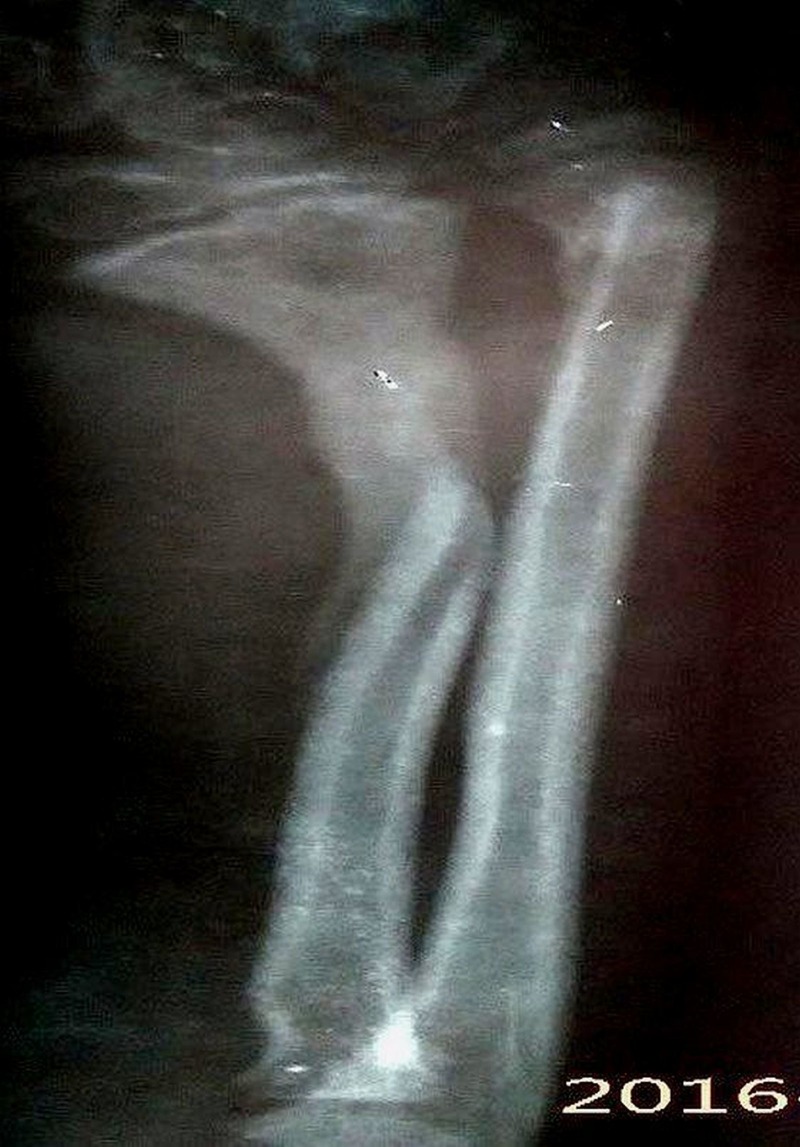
Anteroposterior X-ray of the wrist at eight weeks follow-up showing excellent bone growth in the radial gap.
